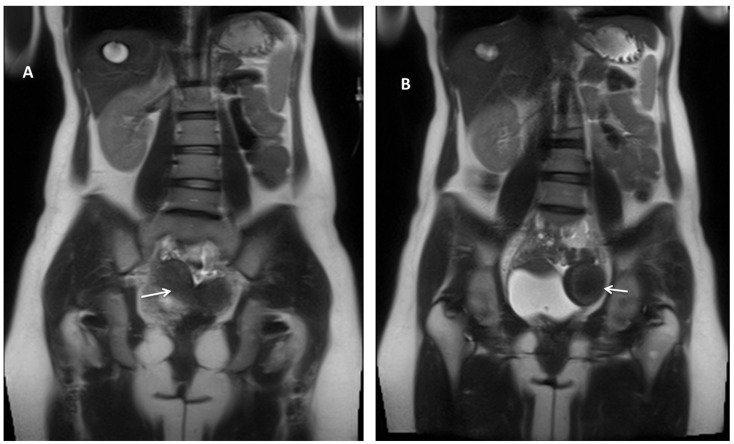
Abdominal and pelvic MRI, coronal T2-weighted images. Pelvic MRI revealed (A) a typical-looking right uterus (white arrow) and right ovary and (B) a pelvic mass located mainly to the left (white arrow) with similar enhancement to the uterus, indicative of a Müllerian duct anomaly type U4aC0V0 with large hematometra and solitary right kidney and absent left kidney.
